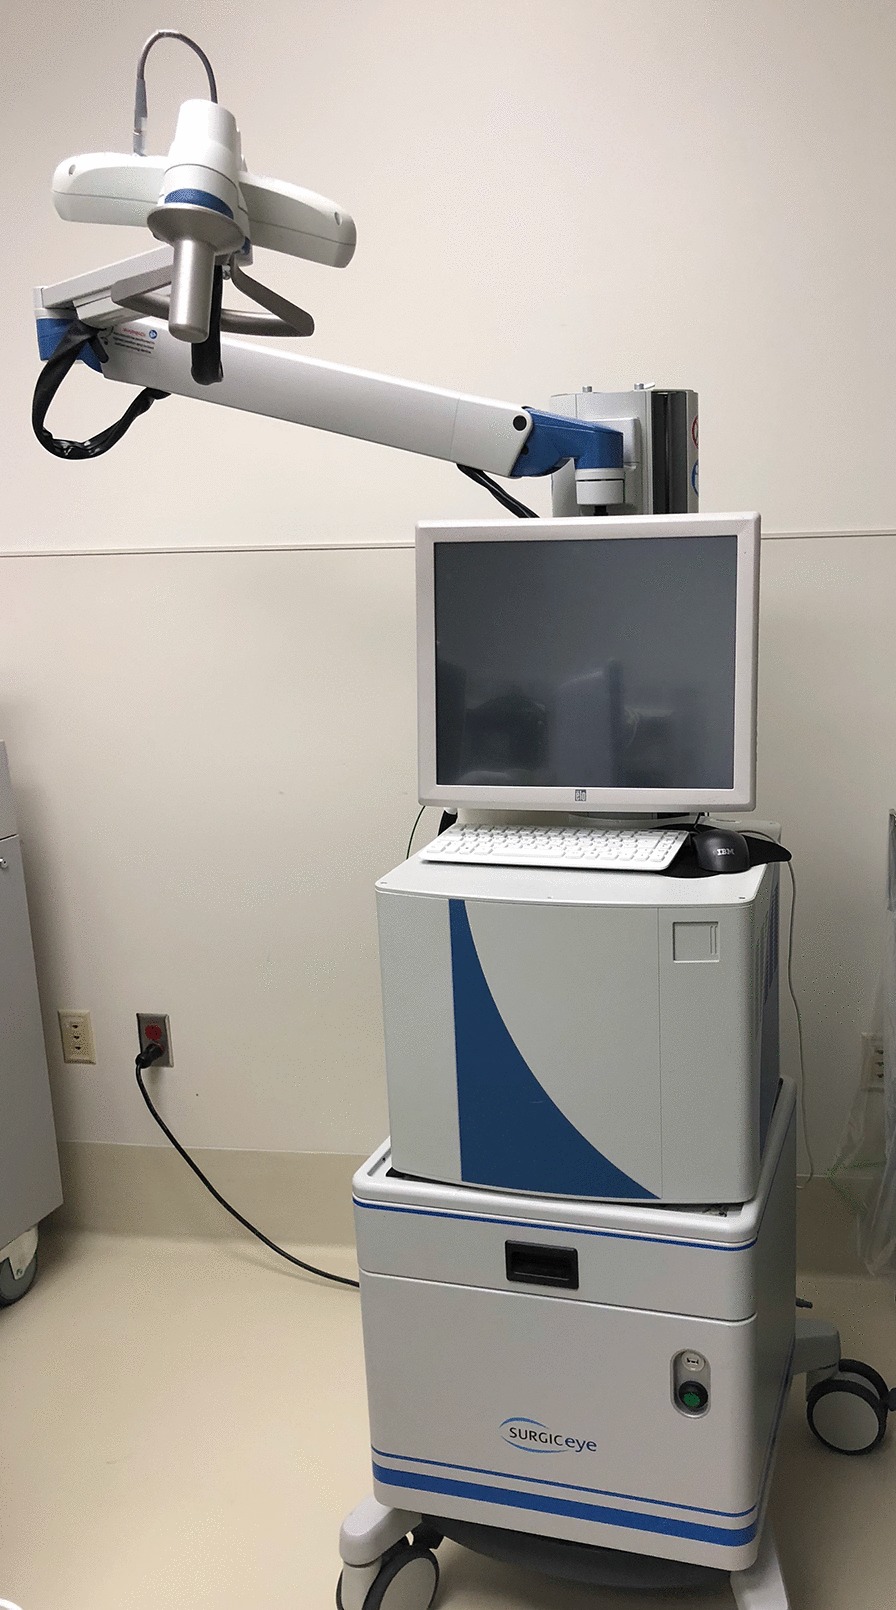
The investigational camera declipse®SPECT system. The SiPM camera is mounted in a holder behind the monitor when not in use
